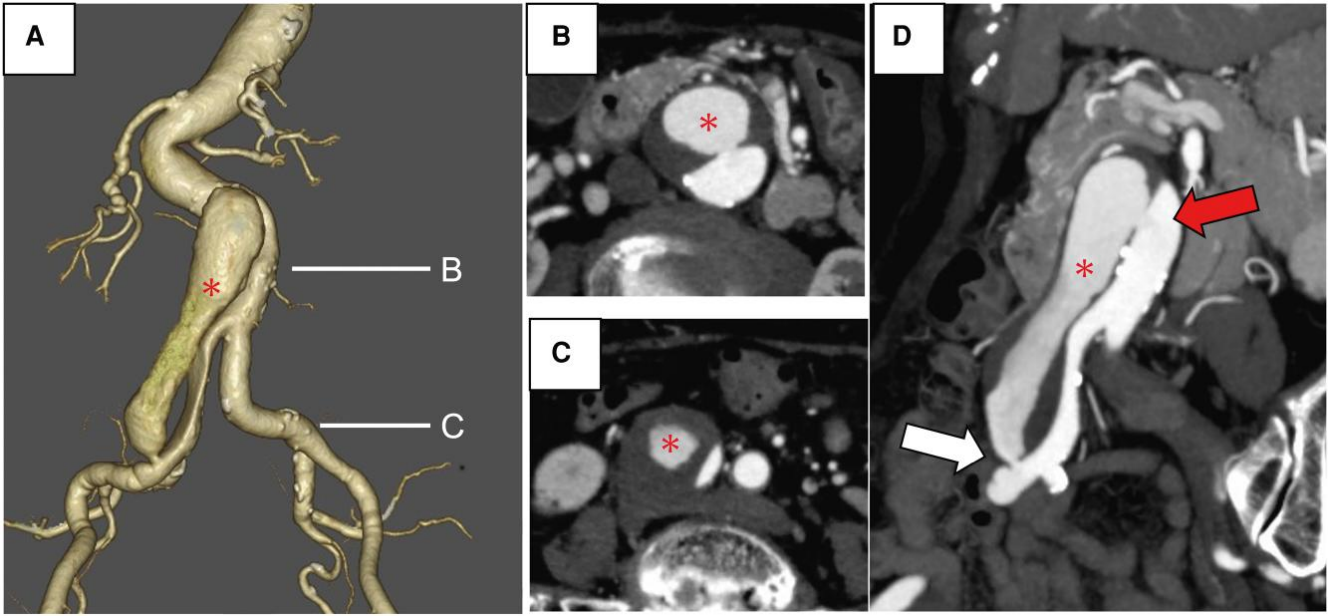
Contrast-enhanced computed tomography of the isolated abdominal aortic dissection. Volume rendering of the abdominal aorta shows a localized false lumen (asterisk) confined to the abdominal region (*A*). Notable findings include stenosis of the right common iliac artery and significant curvature of the infrarenal abdominal aorta. The axial images of the contrast-enhanced computed tomography demonstrate the site of the maximum diameter of the abdominal aortic aneurysm (*B*), the right common iliac artery aneurysm (*C*), and false lumen (asterisk). A 7 mm entry tear in the infrarenal abdominal aorta (superior arrow). Additionally, a 5 mm re-entry tear is noted in the right common iliac artery (inferior arrow) (*D*). CIA, common iliac artery; CT, computed tomography.
